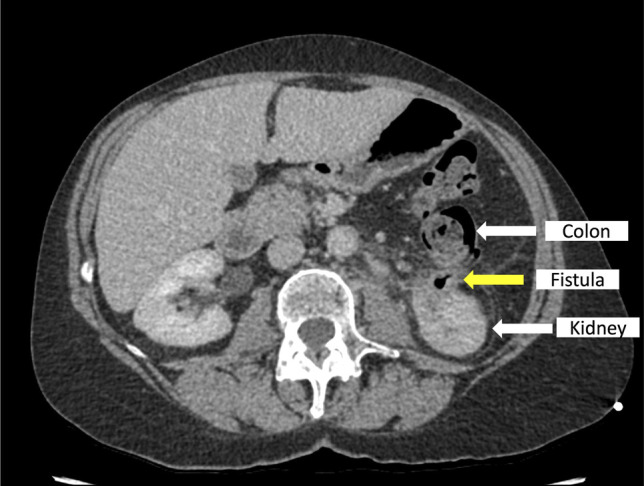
Computed tomography scan demonstrates the left-sided colorenal fistula in February 2024, with air visualized in the fistula tract.
